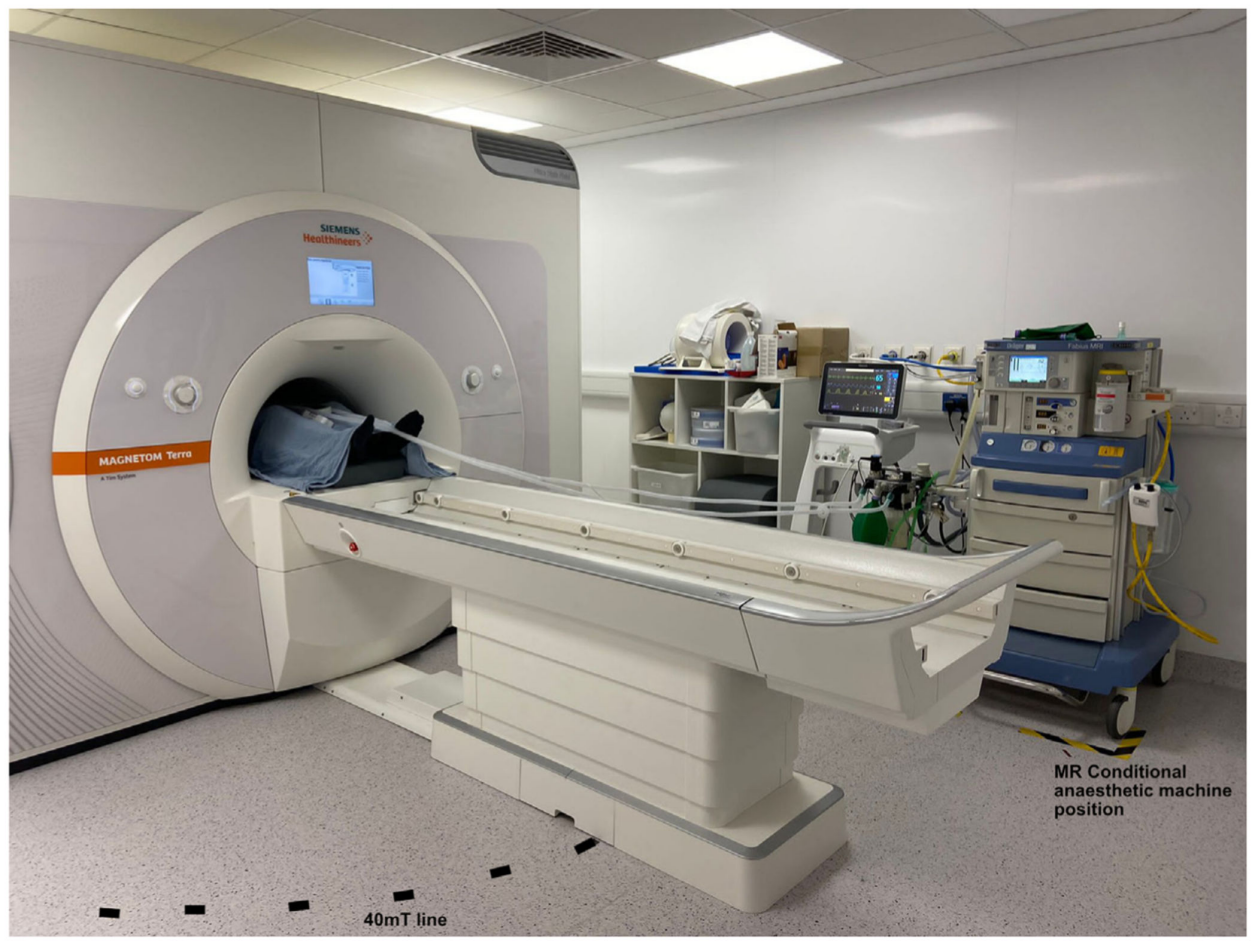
Anaesthetic set-up for ultra-high-field anaesthesia, including an MR conditional Fabius MRI Dräger anaesthesia machine (Dräger, Lübeck, Germany) and Philips Invivo MR400 monitoring system (Philips, Amsterdam, The Netherlands) around a 7T Siemens Magnetom Terra scanner (Siemens AG, Munich, Germany). The black and yellow dashed lines represent where the MR conditional anaesthesia machine should be positioned. The black dashed line around the MRI scanner represents the 40 mT line.
